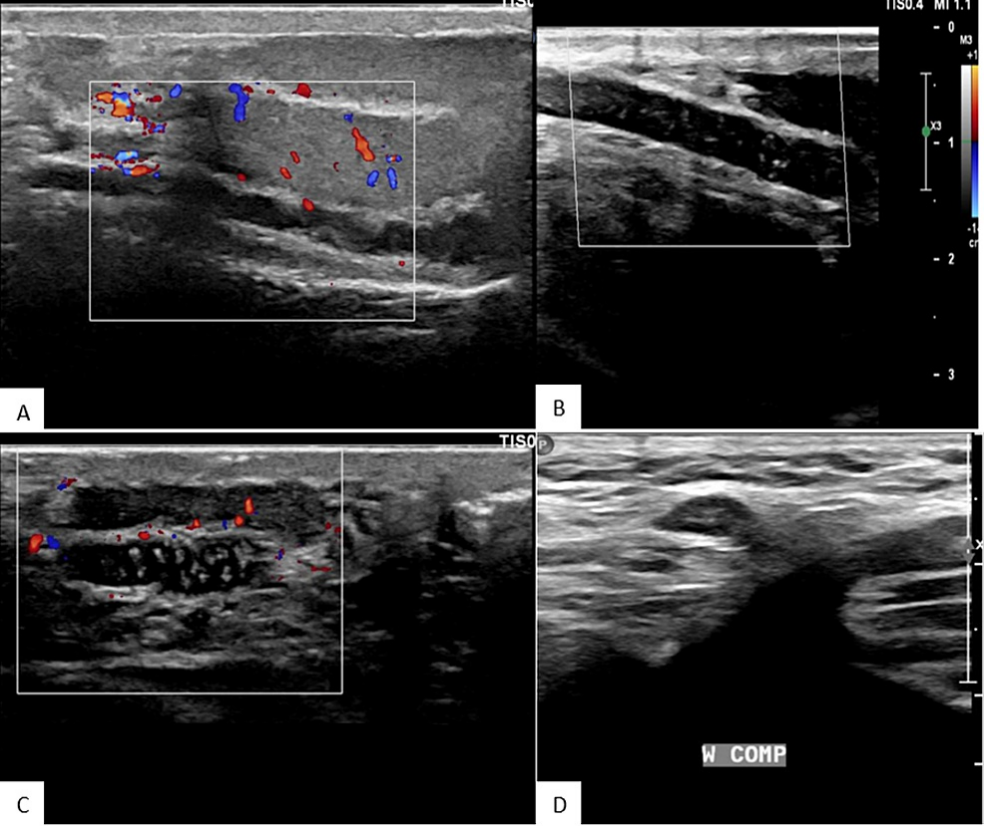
Doppler ultrasound of the testes showing non-compressible (A and B) right and (C and D) left spermatic veins with echogenic thrombus and no flow on Doppler.
